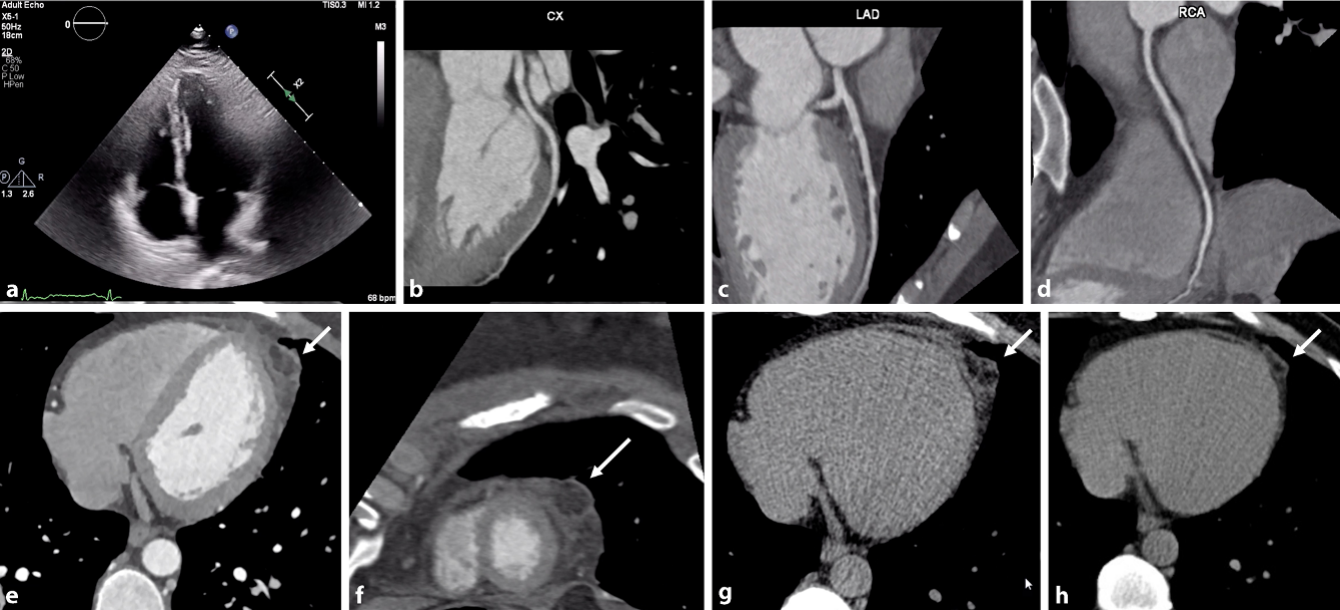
a–h Diagnostic work-up performed to clarify the aetiology of the chest pain. a Apical four-chamber image of the unremarkable transthoracic echocardiogram performed at admission. b–d Coronary computed tomography angiography (CCTA) images of the circumflex coronary artery (*CX*), left anterior descending coronary artery (*LAD*) and right coronary artery (*RCA*). e–g CCTA images showing a lesion (22 mm × 15 mm) located anterior to the left ventricle (*arrows*), suggestive of epipericardial fat necrosis (EFN); the less common CT pattern of EFN is shown here, comprising an ovoid fat mass with dense stranding. h Re-evaluation image showing almost complete resolution of the EFN (*arrow*)
